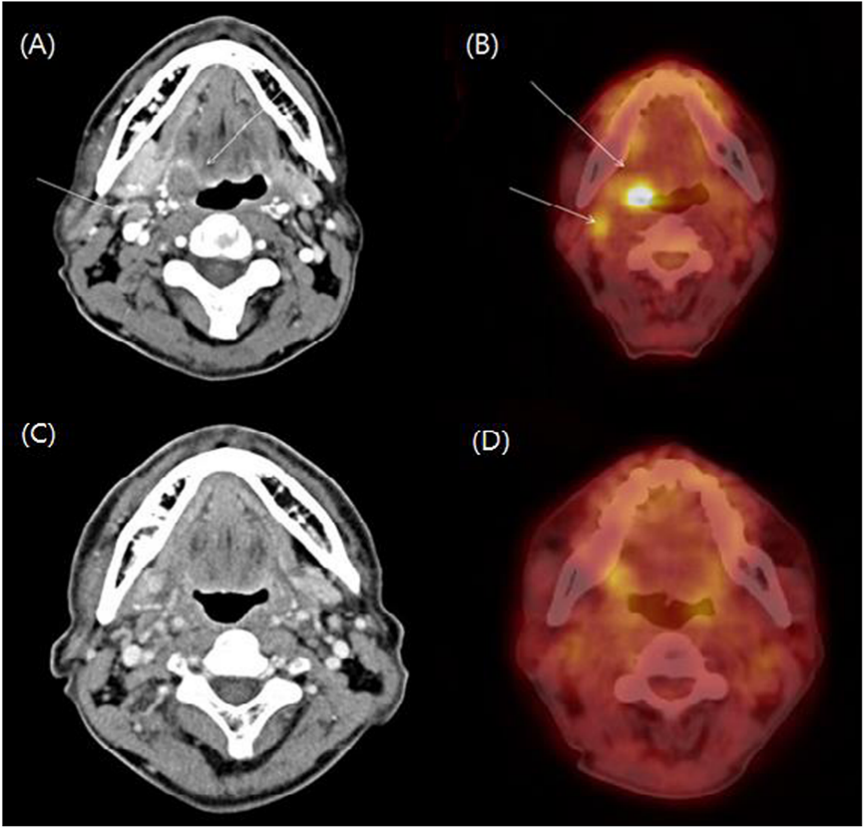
F-18 FDG PET and CT scan findings. (A) Ill defined heterogeneous enhancing soft tissue mass lesion in the right tongue base and enlarged lymph nodes in the right level II are observed on a plain contrast CT scan. (B) F-18 FDG PET reveals a hypermetabolic lesion (arrow) in the right tongue base and lymph node in the right level II. (C) A follow-up CT scan reveals no evidence of mass lesion in the right tongue and no significant lymph node in both necks after the treatment. (D) After the treatment, a 1 month follow-up PET scan shows disappearance of the previous hypermetabolic lesion.
